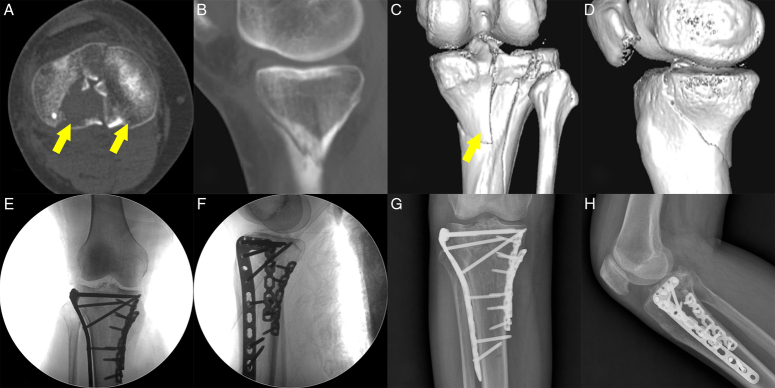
The therapeutic protocol for PL+PM fractures. (A–D) Preoperative CT images reveal a posterior bi-quadrant fracture with a PLF of more than one-third of the lateral articular area and a large posteromedial split fragment can be observed. (E,F) Rigid fixation is achieved by a lateral buttress-rafting plate to maintain the reduction of the PLF, and two reconstruction plates for the antiglide effect to stabilize the large split fragment of the PM column. (G,H) Postoperative 3-month X-rays demonstrate excellent reduction, even during flexion. PL, posterolateral; PM, posteromedial; PLF, posterolateral tibial plateau fracture.
